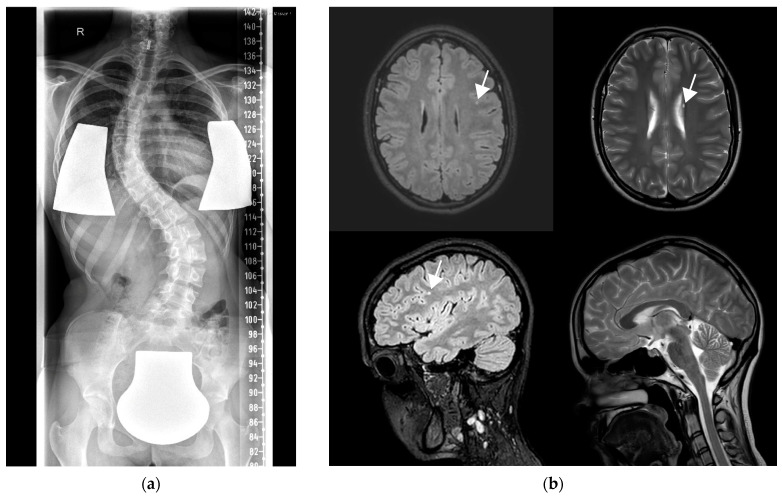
(a) X-ray showing scoliosis in patient 2 at the age of 17 years. (b) Brain MRI in patient 2 at the age of 17 years with left frontal FLAIR hyperintensity (upper left axial and lower left sagittal images), periventricular cyst (upper right axial) but no other abnormalities (lower right sagittal).
